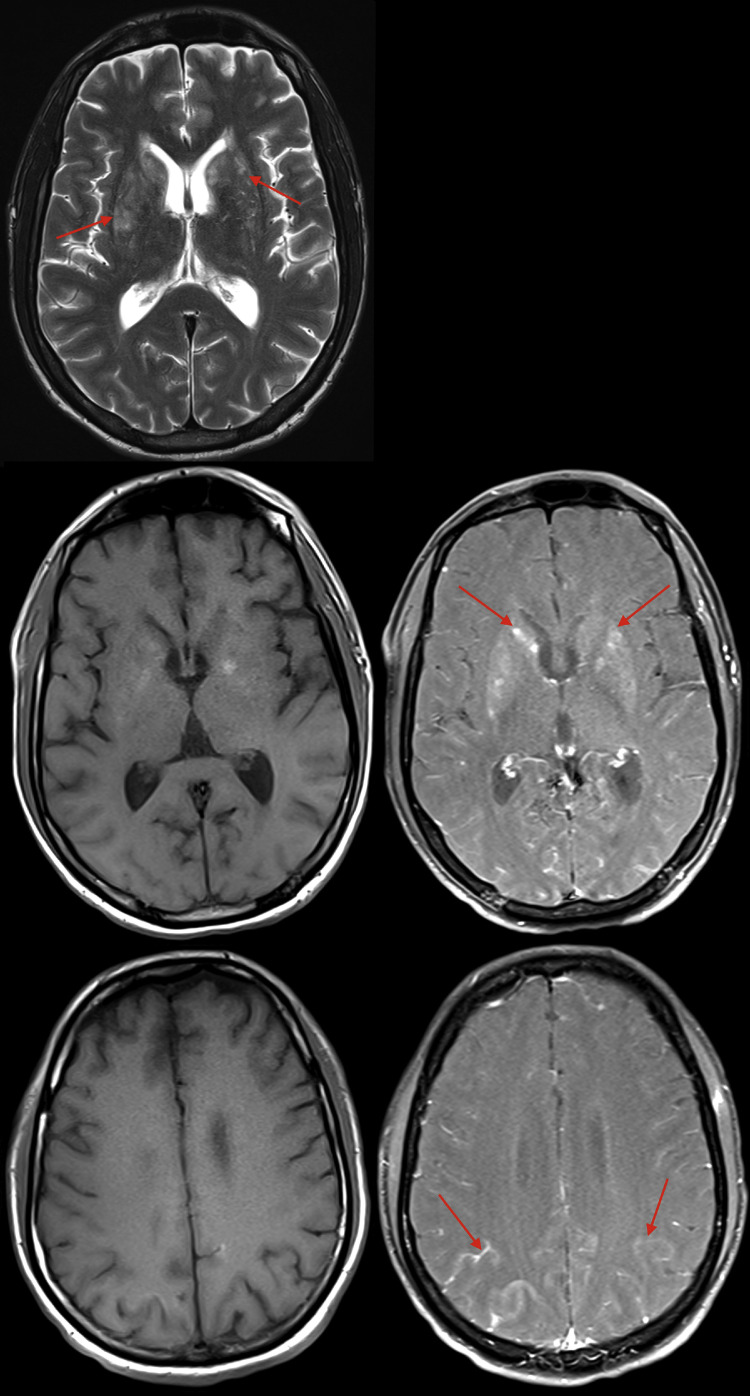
Axial T2-weighted MRI sequence (A) demonstrates hyperintensities involving the bilateral corpus striatum (red arrows). Axial T1-weighted sequences pre- (B) and postcontrast (C) demonstrate multifocal nodular enhancement involving the bilateral corpus striatum (red arrows); these lesions demonstrate intrinsic T1 hyperintensity. Additionally, axial T1-weighted sequences pre (D) and postcontrast (E) demonstrate leptomeningeal enhancement involving the bilateral frontal and parietal lobes.
